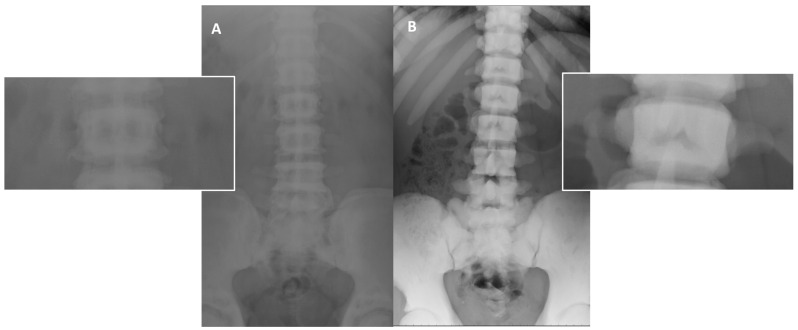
Different pattern of bone sclerosis in different type of osteopetrosis. Conventional radiographies (AP projection) in osteopetrosis ADO type 2 (A) and ARO (B) show different type of abnormally increased bone density: well-defined sclerosis of vertebral endplate (‘rugger jersey spine’—(A)) in ADO type 2, and complete and marked sclerosis of the whole vertebrae in ARO type (B).
